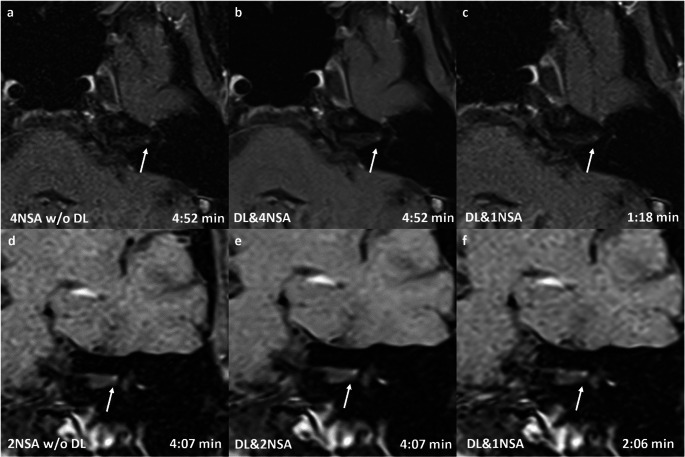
0.55 T MRI of 55-year-old female patient with punctual vestibularis schwannoma (VS) (maximum diameter 1mm) on the left site (arrow). All reconstructions without (w/o DL) and with (DL) deep learing based denosing algorithsm and with different number of averages (NSA) in transversal (a-c) and coronal (d-f) orientation) clearly show the enhancing lesion on the left site. The VS conspicuity of the transversal sequences was rated highest for DL&4NSA (Likert scale: 5), followed by DL&1NSA (Likert scale: 4) and DL w/o DL (Likert scale: 4). The quality of the coronal images was rated highest for DL&2NSA (Likert scale: 5), the other reconstructions received an equal rating (Likert scale 4)
